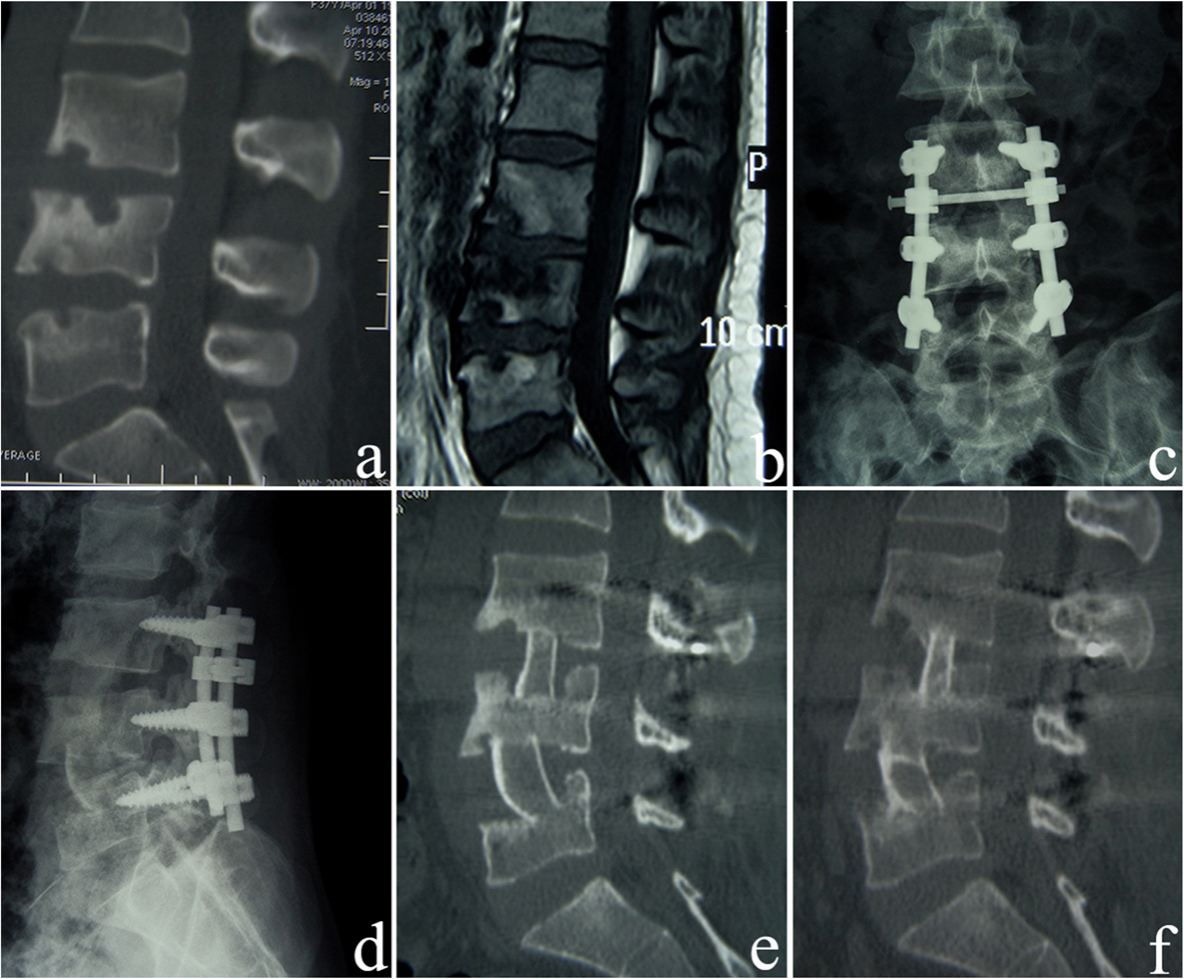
A 34-year-old female patient underwent anterior-posterior surgery and affected-vertebrae fixation. a The preoperative sagittal CT reconstruction image shows destruction of L3, L4, and L5 vertebrae and narrowing of the L3-L4 intervertebral space. b The preoperative sagittal contrast-enhanced MRI shows destruction of L3, L4, and L5 vertebrae and destruction of the L3-L4 and L4-L5 intervertebral disks. c, d One month after surgery, the anteroposterior and lateral X-ray images show that the fixation of the affected vertebrae is excellent. L3 and L4 vertebras are fixed with short pedicle screws. e Three months after surgery, the sagittal CT reconstruction shows that the lesion in the L3, L4, and L5 vertebrae has debrided completely, and the iliac bone grafts are firm. f Five years after surgery, the sagittal CT reconstruction shows that the L3–5 tuberculosis lesions are cured, and the bone graft fusion is solid
